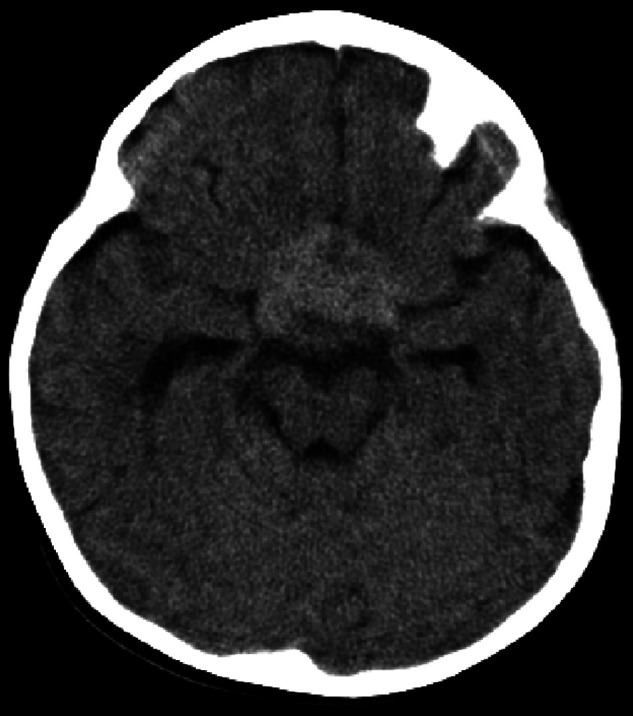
CT scan of the brain (case 1) revealed a large hyperdense suprasellar lesion and mild ventricular dilatation.
